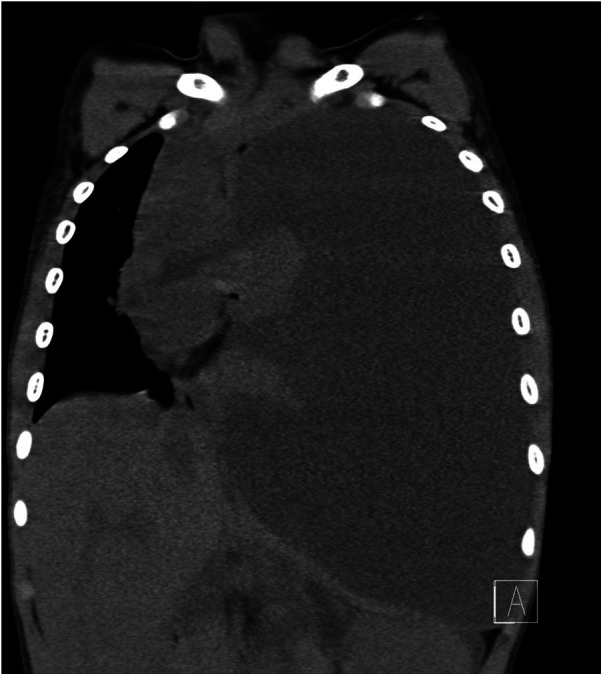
Computed tomography indicating large left pleural effusion.
